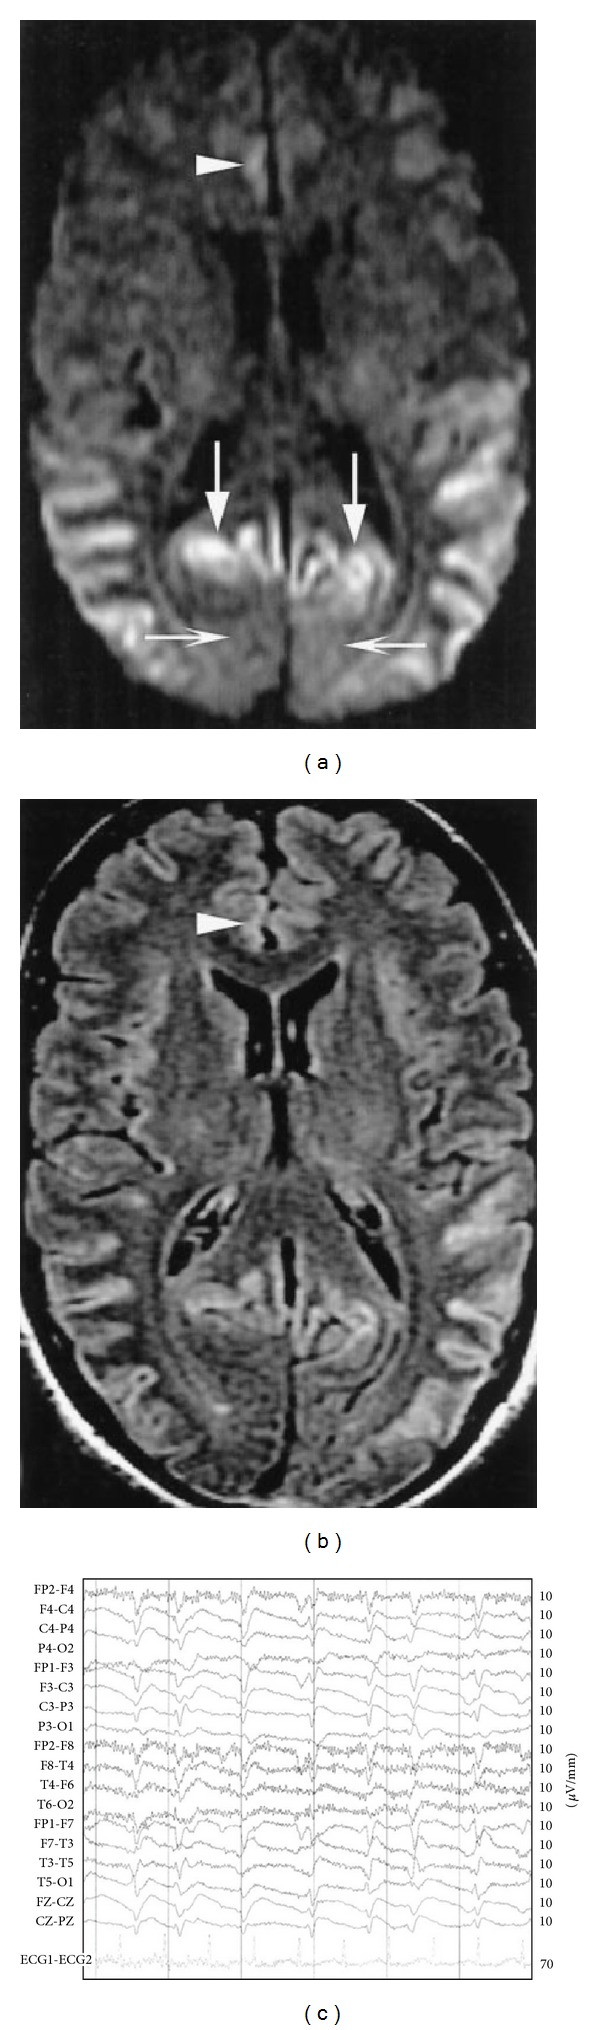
(a) A 50-year-old man with definite sCJD. Axial DWI shows pathologic hyperintensity in bilateral posterior temporoparietal neocortex. Cortex along parietal-occipital fissure is abnormally hyperintense (vertical arrows), but primary visual region is spared (horizontal arrows). Note asymmetric abnormal hyperintensity in right cingulum (arrowhead). Striatum is uninvolved. (b) FLAIR image at same level shows more subtle pathologic hyperintensity in all abnormal regions on DWI, as shown in cingulate cortex (arrowhead) [10]. (c) Definite sCJD (MM1); total duration: 10 months; EEG at 6 weeks: typical (used to classify case as probable); source: http://www.eurocjd.ed.ac.uk.
